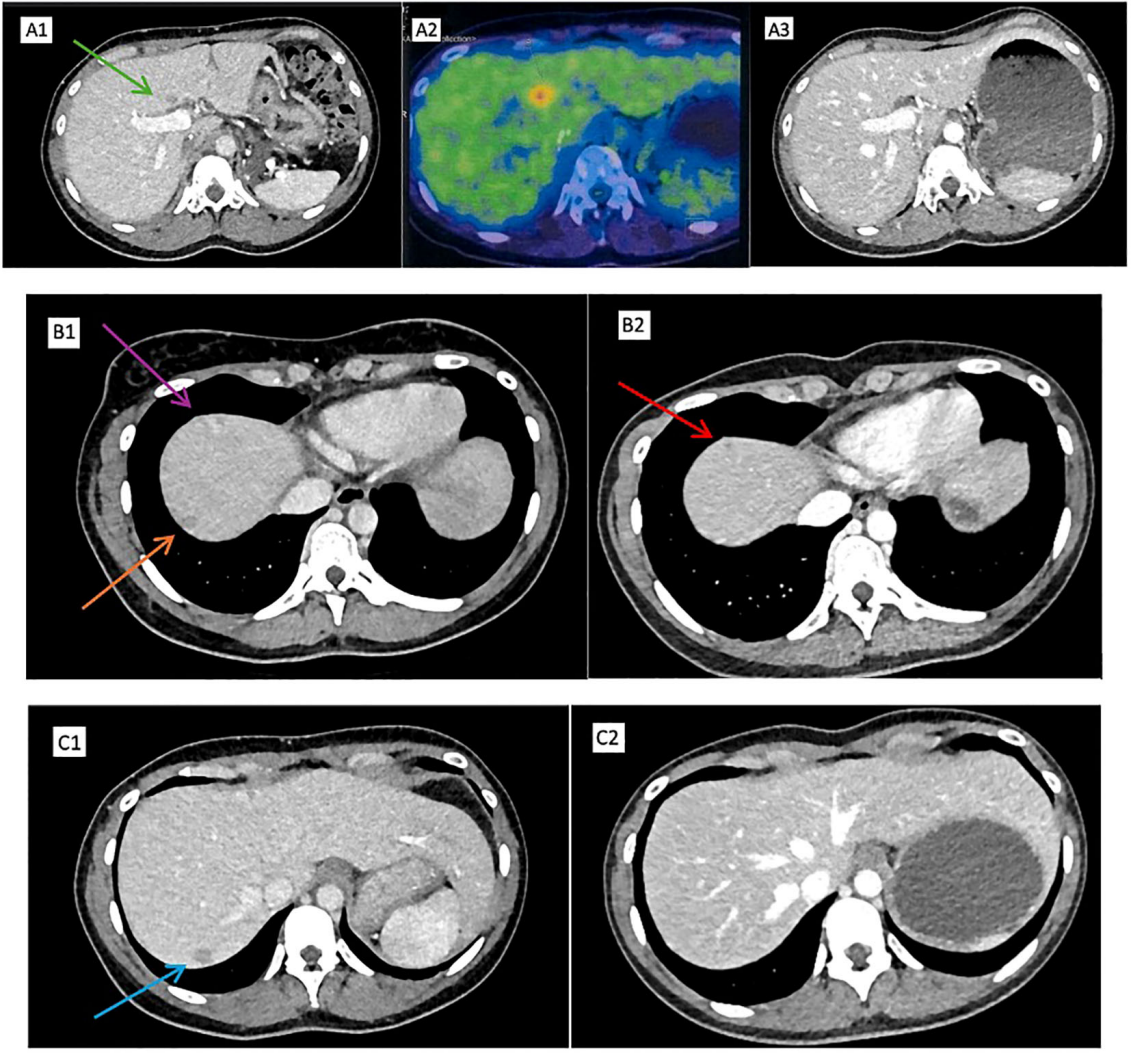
Positron emission tomography (PET)–computed tomography (CT): (A1) Hypodense lesion in segment 4 (green arrow). (A2) FDG-avid irregular hypodense liver lesion at segment 4 (SUVMax 5.1) after cycle 1. (A3) Complete resolution of hypodense liver lesion in segment 4 after cycle 4. (B1) Hypodense liver lesions in segment 8 (purple arrow) and segment 7 (orange arrow). (B2) Smaller hypodense liver lesion in segment 8 after cycle 4 (red arrow). (C1) Hypodense liver lesion in segment 7. (C2) Complete resolution of hypodense liver lesion in segment 7 after cycle 4.
